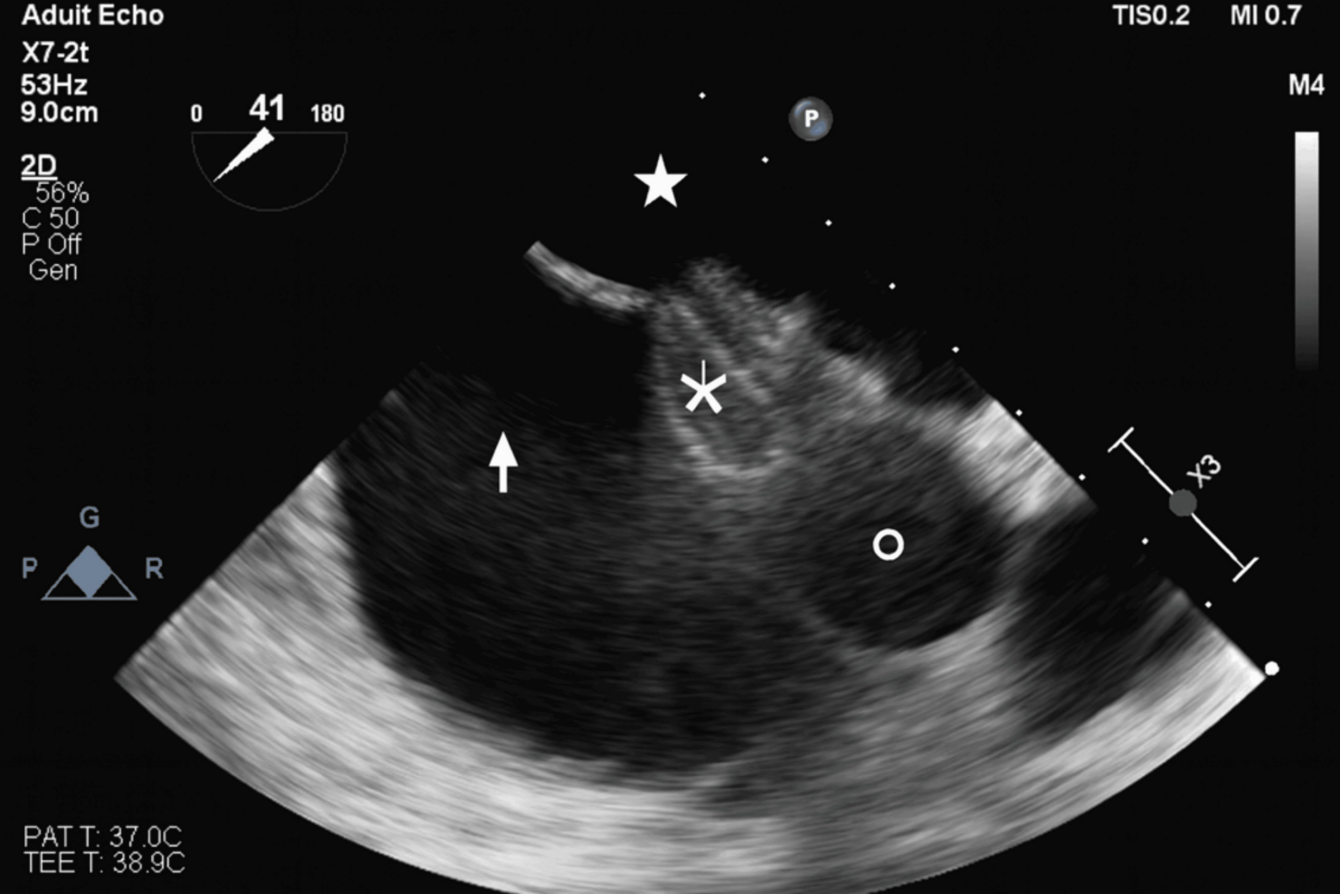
Intra-procedural TOE demonstrating correct PFO occluder deployment Arrow indicated right atrium, solid star indicates left atrium, snowflake indicates PFO occluder, and circle indicates aortic root. TOE - transesophageal echocardiography; PFO - patent foramen ovale
